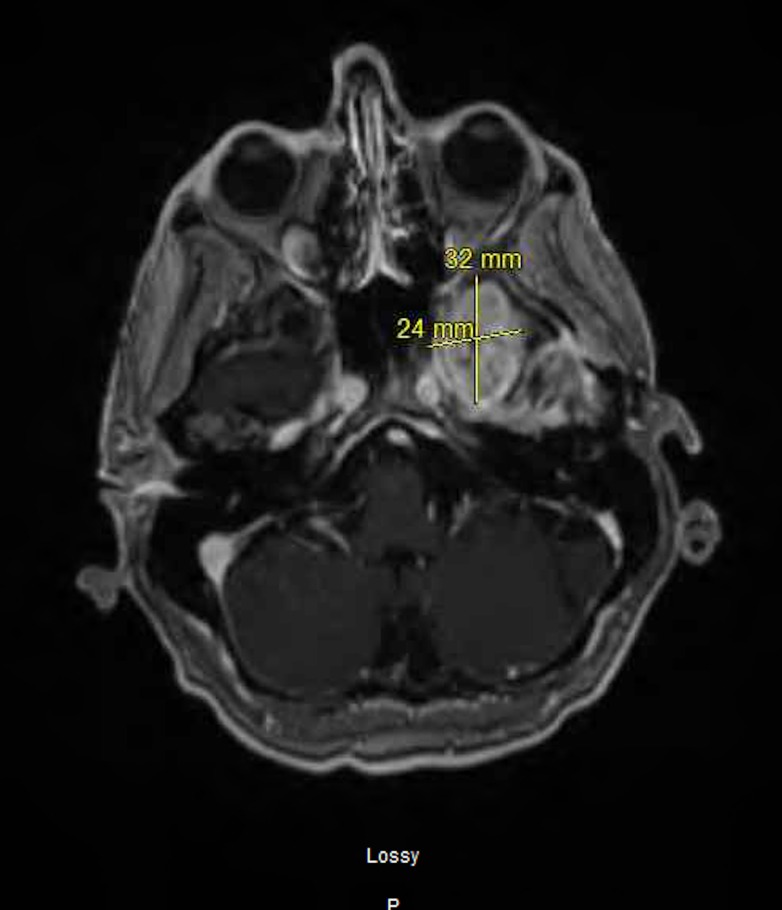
Brain MRI, Axial View with Contrast Enhancement A large temporal lobe mass bridging the skull base into the left pterygomaxillary region.
